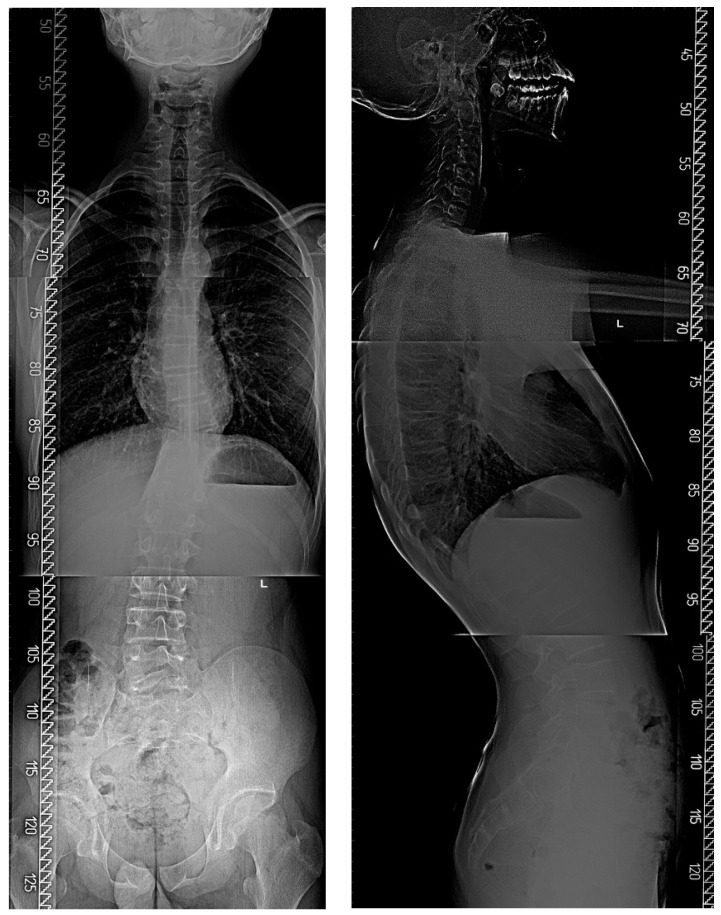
Standing radiographs of the entire spine of a 15-year-old boy with a progressive congenital spinal deformity.
